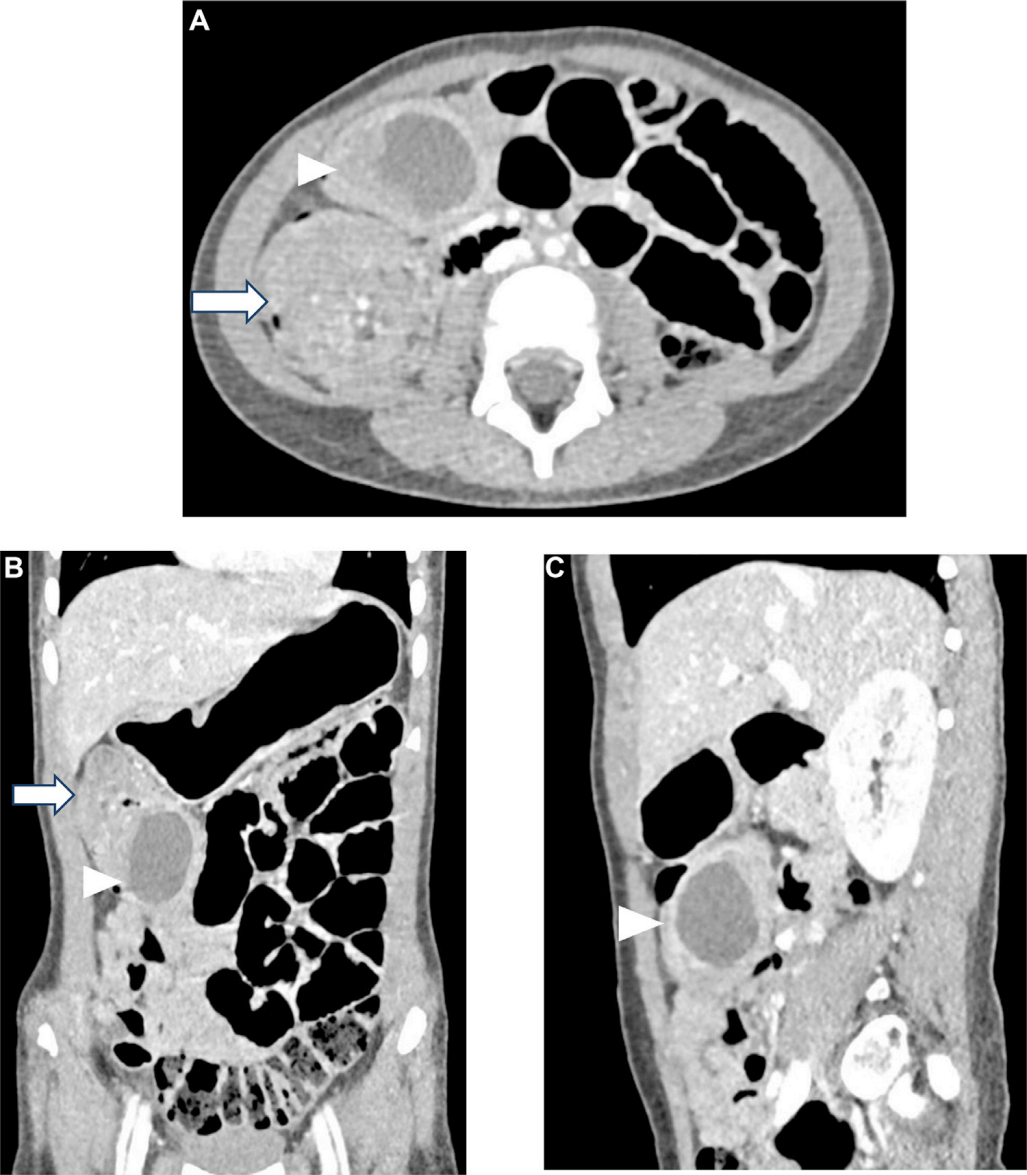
(A, B and C) Contrast-enhanced CT images of the abdomen in axial (A), coronal (B), and sagittal (C) planes demonstrate ileocolic intussusception characterized by a segment of bowel telescoping into the adjacent distal bowel, seen as an irregular, thickened bowel wall with layered enhancement (white arrows). Adjacent to this, a well-defined, low-attenuation cystic lesion with a thin, smooth wall is visualized (white arrowheads). This cystic structure corresponds to the cecal duplication cyst, lying close to the ileocecal region and distinct from the intussuscepted bowel segment.
